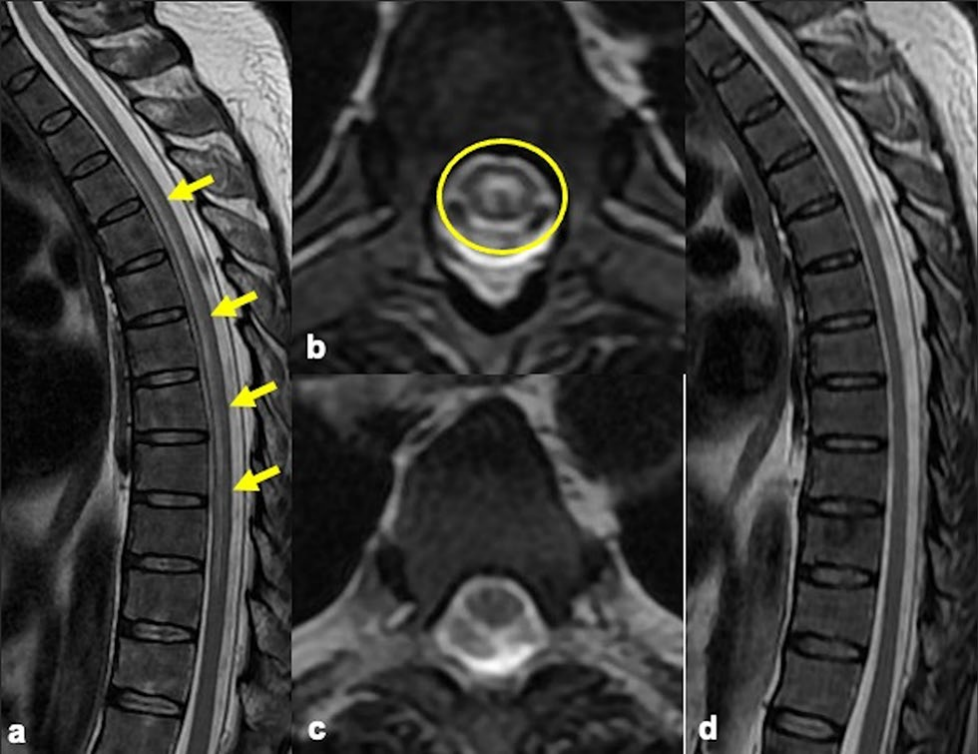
Sagittal and axial T2 MRI weighted images (a, b) show longitudinally extensive myelitis (arrows) involving the central gray matter with an “H shape” appearance on axial images (circle). Axial and sagittal T2 follow-up MRI (c, d) demonstrates resolution of the abnormal high T2 signal
